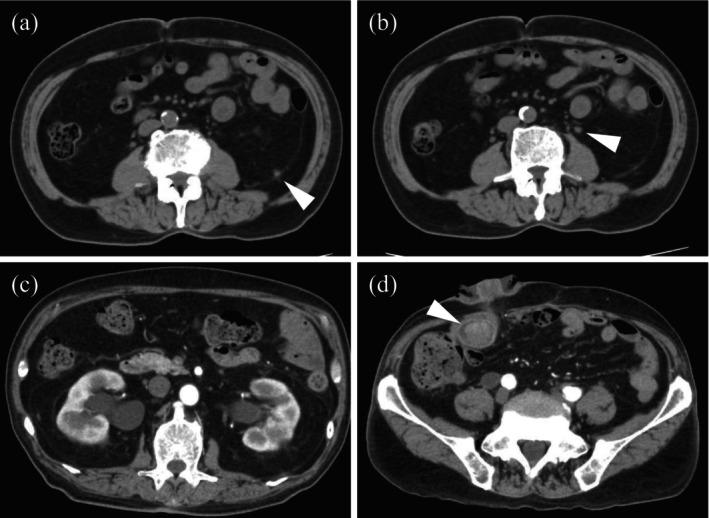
Horizontal view of computed tomography images. (a, b) Axial computed tomography scan shows two of multiple metastatic lesions (arrowhead) located in the retroperitoneum. (c) Contrast‐enhanced computed tomography shows bilateral hydronephrosis. (d) A mass (arrowhead) is visualized within the ileal conduit.
